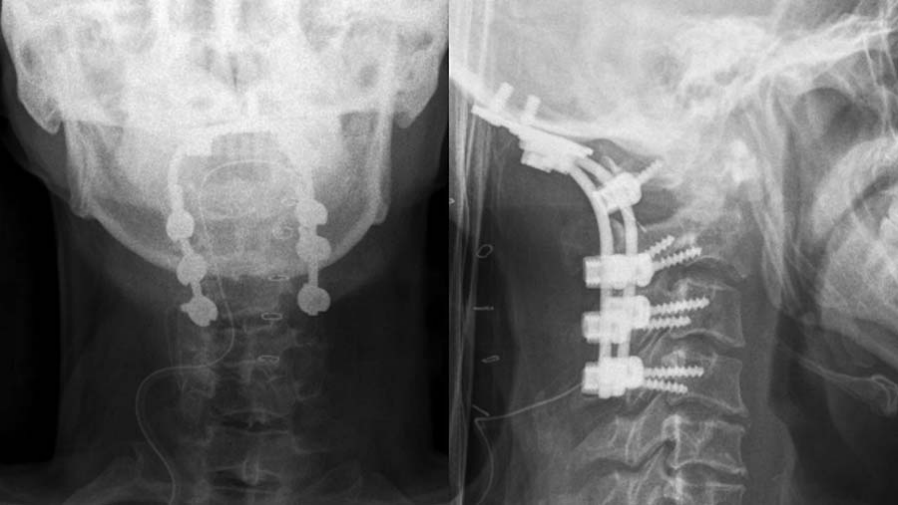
Postoperative x-ray showed proper occipitocervical stabilization.
